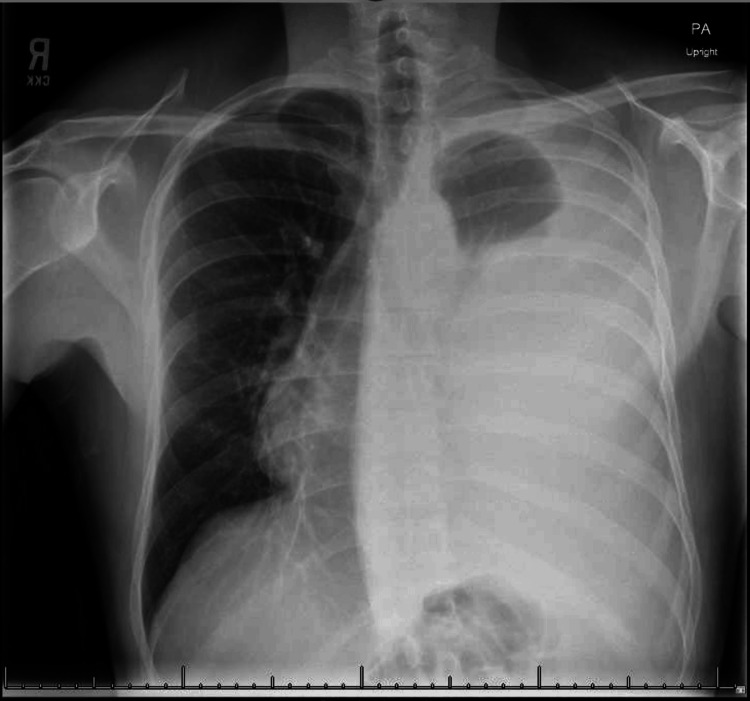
Chest radiograph demonstrating a left-sided massive pleural effusion causing near-total opacification of the left hemithorax and a rightward mediastinal shift.
